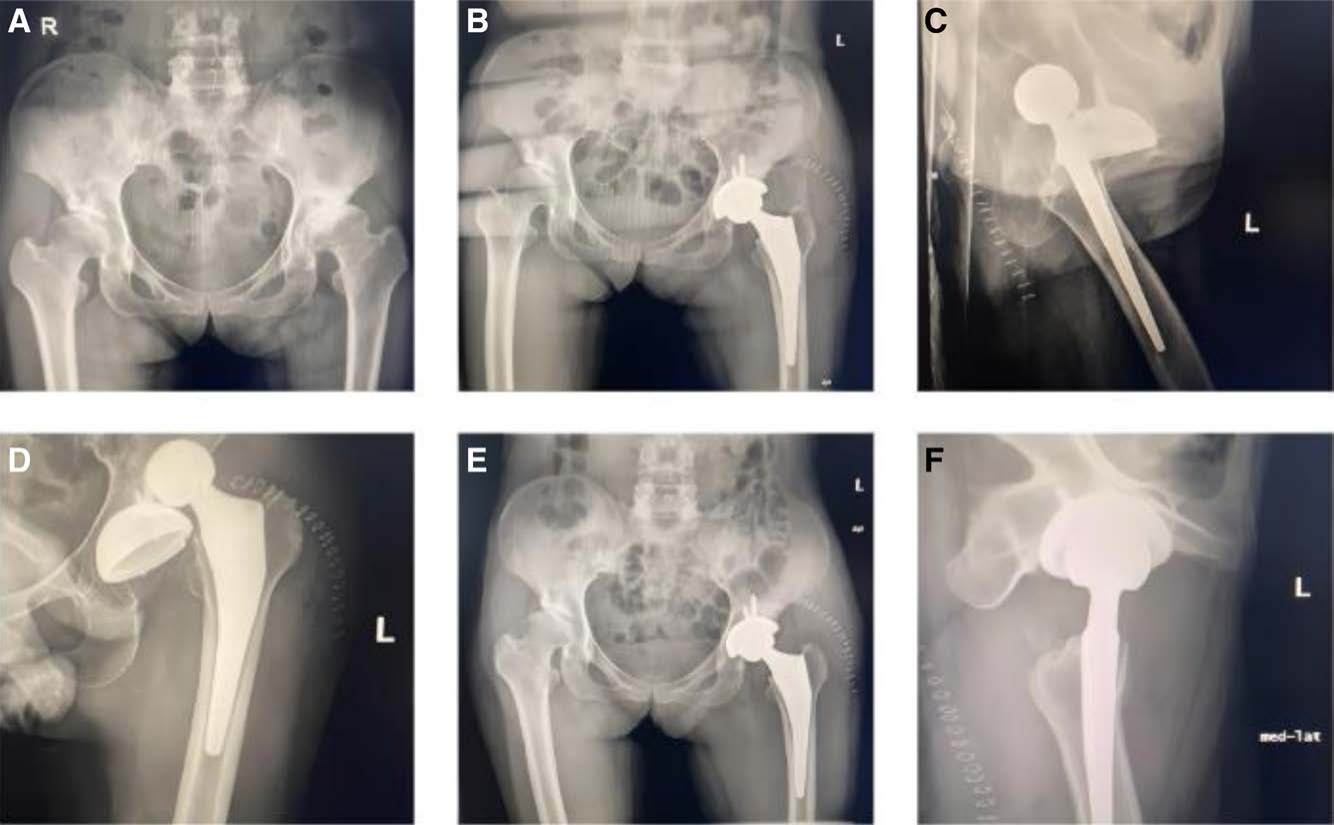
Prosthetic dislocation for a 56-yr-old female patient. (A) Preoperative pelvis X-ray. (B) Preoperative pelvis X-ray. (C, D) Anterior dislocation hip X-ray after surgery. (E) Pelvic X-ray after manual reduction surgery. (F) Hip X-ray after manual reduction surgery.
